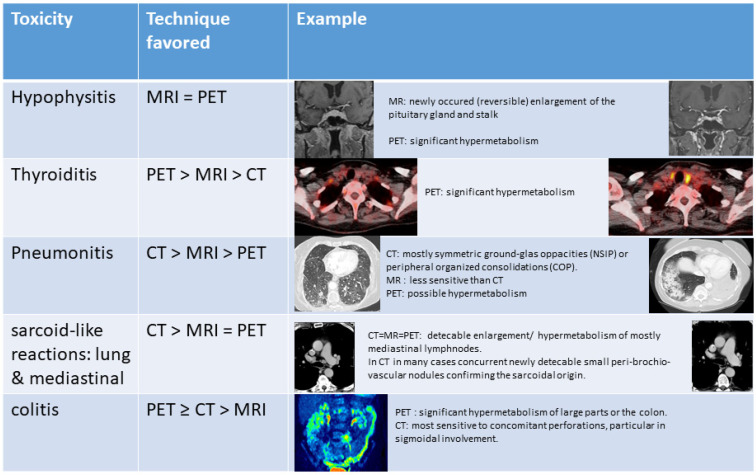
Preferred imaging modality for the characterization of immune-related side effects (PET refers to ^18^F-FDG-PET).
